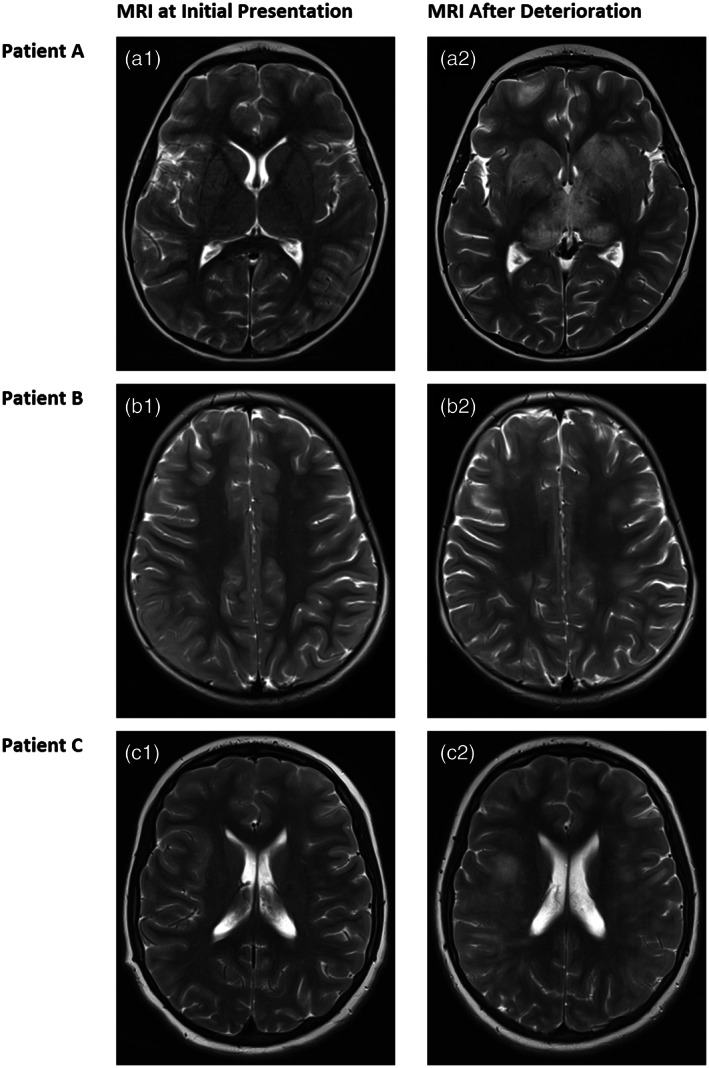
Comparative T2 MRI images in initial presentation (a1, b1 and c1) and after deterioration (a2, b2 and c2) of patient A (a1 and a2), patient B (b1 and b2) and patient C (c1 and c2). Initial MRI images are normal or showing subtle changes only with repeat MRI showing clear inflammatory changes in the deep grey and white matter regions. Axial brain MRI images after deterioration show multifocal, poorly defined T2 high signal in deep grey matter of patient A, multifocal, poorly defined T2 high signal throughout the white matter and cortex in patient B and white matter T2 high signal in patient C. MRI, magnetic resonance imaging.
